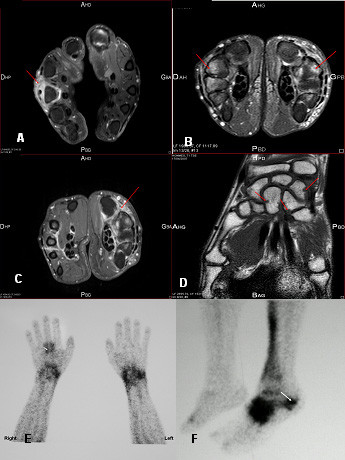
Magnetic resonance imaging (MRI) and bone scintigraphy of the hands and wrists of a 60-year-old man with chikungunya virus infection revealing. A. Arthritis of the 3^rd ^metacarpo-phalangeal joint of the right hand with extensor tenosynovitis associated with intra-articular swelling (red arrow on axial section, time-resolved contrast-enhanced T1-weighted sequence after Gadolinium injection with fat suppression) B. Bilateral periostum inflammation and oedematous carpitis with synovitis predominating on the left hand (arrow on axial section, time-resolved-enhanced T2-weighted sequence with fat suppression) C. Asymmetric inflammatory carpitis with multiple synovitis of flexors of the left wrist (red arrows on axial section, time-resolved contrast-enhanced T1-weighted sequence after Gadolinium injection with fat suppression) D. Bone destruction on the left hand (red arrows on coronal section, time-resolved contrast-enhanced T1-weighted sequence with fat suppression) E. Bone scintigraphy of the wrists and hands showing an intense focus of technetium 99 m-labeled methylene disphosphonate tracer uptake, particularly in the right metacarpo-phalangeal wrist. F. Bone scintigraphy of the left foot showing an intense focus of technetium 99 m-labeled methylene disphosphonate tracer uptake, particularly in the left ankle.
